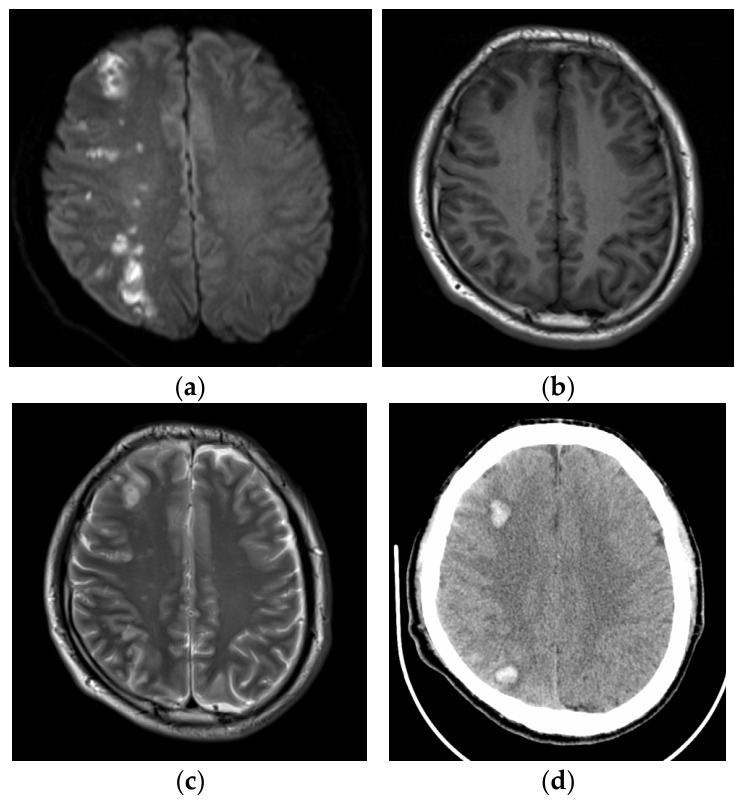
Imaging findings of cerebral infarction with hemorrhagic transformation after hyaluronic acid injection: (a) DWI shows multiple acute infarcts in the right frontal–parietal lobe; (b) T1-weighted image shows slightly prolonged T1 signal in the right frontal–parietal lobe; (c) T2-weighted image shows slightly prolonged T2 signal in the right frontal–parietal lobe; (d) CT shows hemorrhage in the right frontal–parietal lobe.
